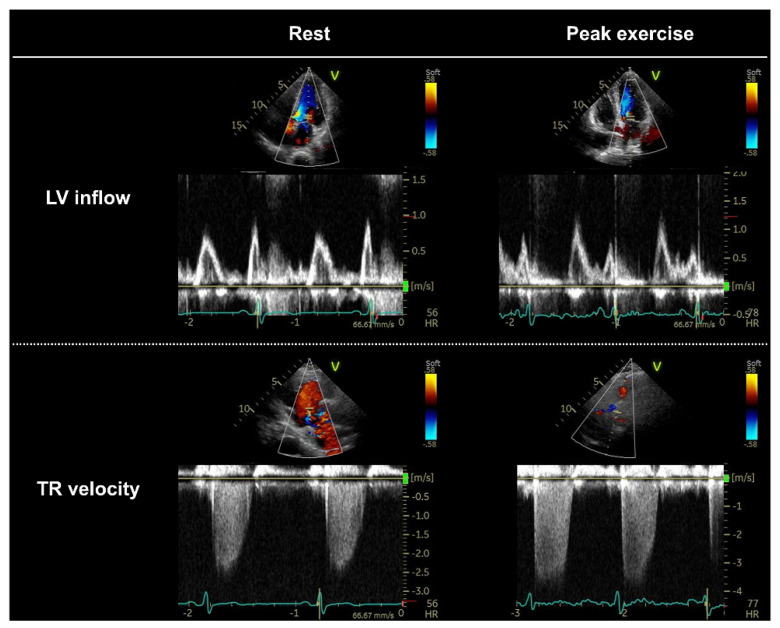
Key parameters in diastolic stress echocardiography. A 72-year-old woman who presented with exertional dyspnea was referred for diastolic stress echocardiography. She had a normal ejection fraction (61%), slightly elevated B-type natriuretic peptide (NP) levels (48.2 pg/mL), borderline E/e′ ratio (10.9), and a normal tricuspid regurgitation (TR) velocity (2.4 m/s). During peak exercise, the E wave and E/e′ ratio increased (16.9), with a concomitant elevation in TR velocity (3.8 m/s). LV, left ventricular.
